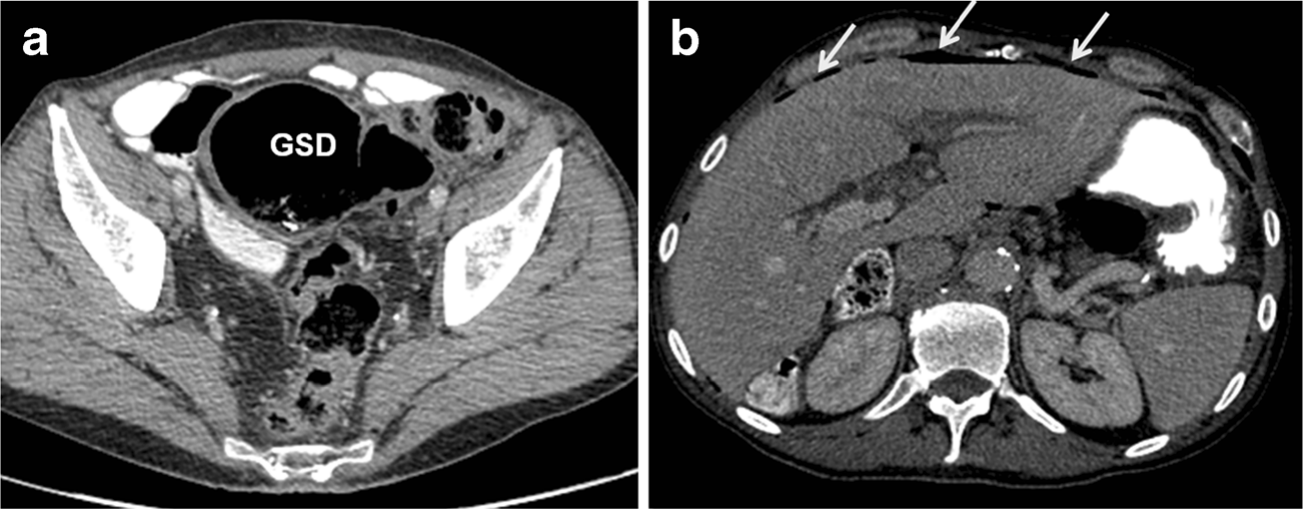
A 63-year-old man with giant sigmoid diverticulum (GSD) presented with acute abdomen. Axial contrast-enhanced CT images through the pelvis (a) and upper abdomen (b) show the GSD complicated with acute diverticulitis, perforation, and pneumoperitoneum (arrows)
